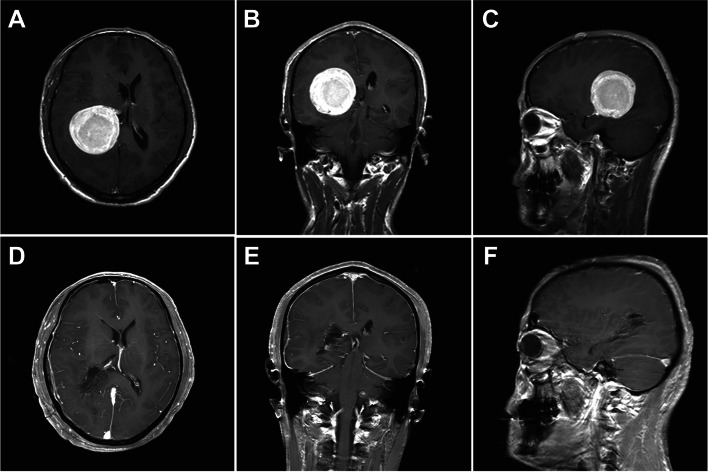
Ventricular meningioma resection via the posterior-temporal keyhole approach. A–C Pre-operation MR images. D–F postoperation MR images
